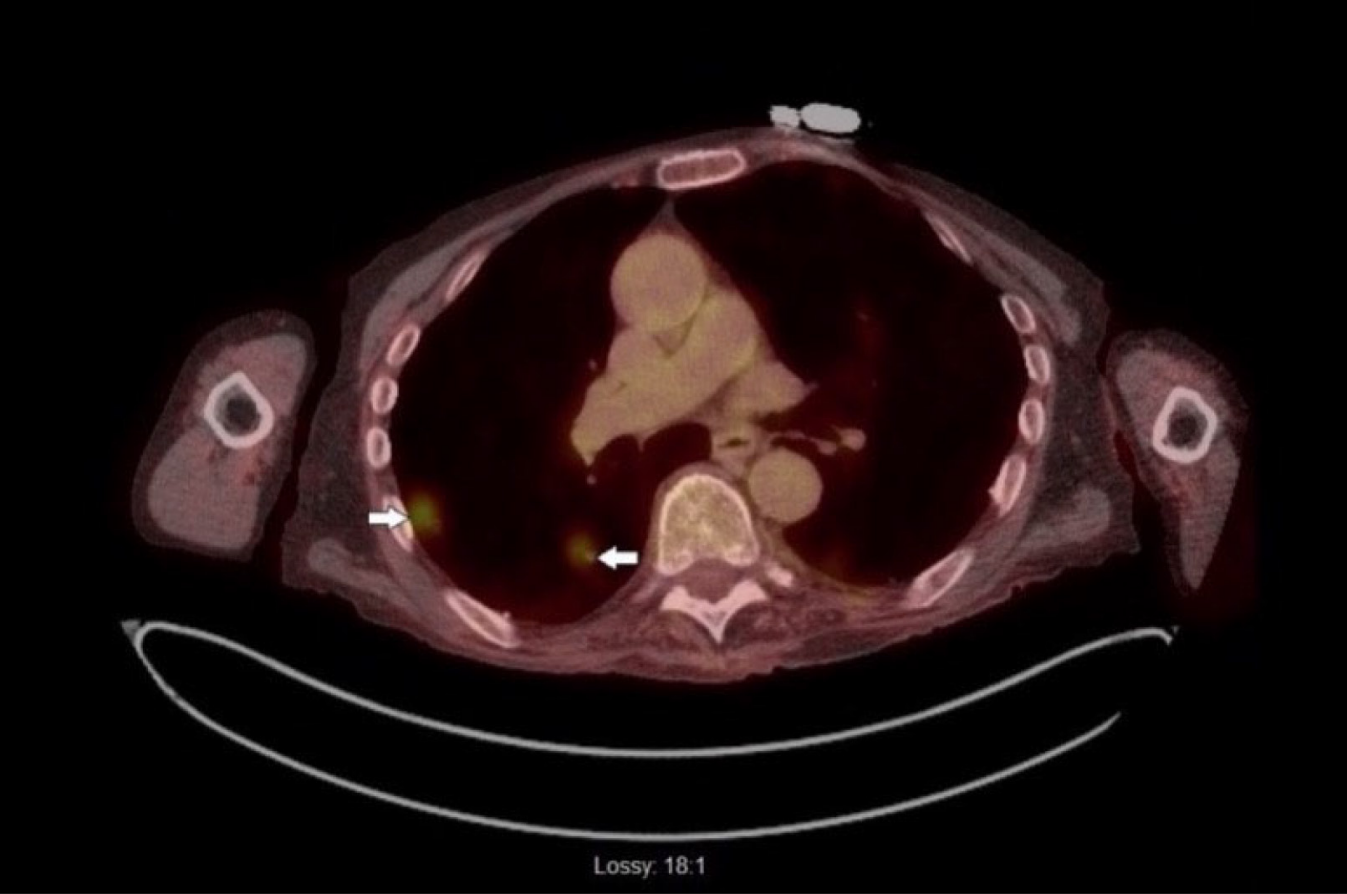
PET CT demonstrating innumerable FDG avid pulmonary nodules.
